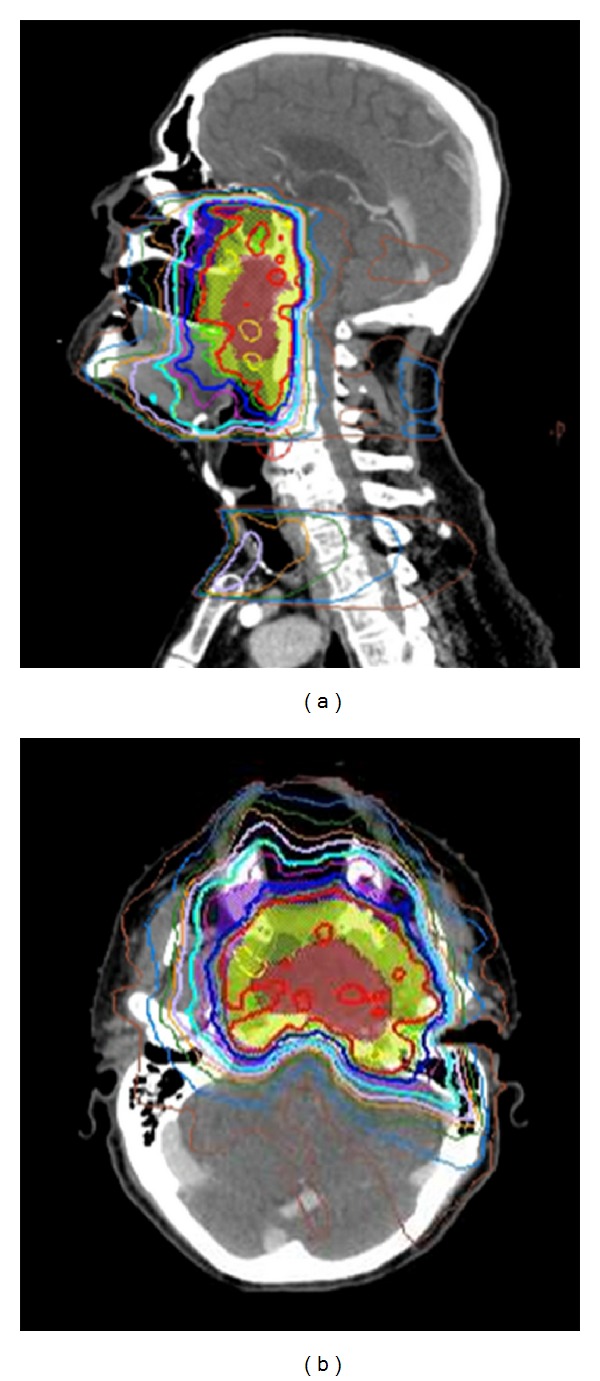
IMRT plan for the same patient above with a locally advanced nasopharyngeal cancer demonstrating sharp dose gradient at the level of the skull base, clivus, and upper cervical spine in close proximity to temporal lobe brain, brainstem, and spinal cord. CBCT was used for daily alignment to ensure setup accuracy (see Figure 3).
